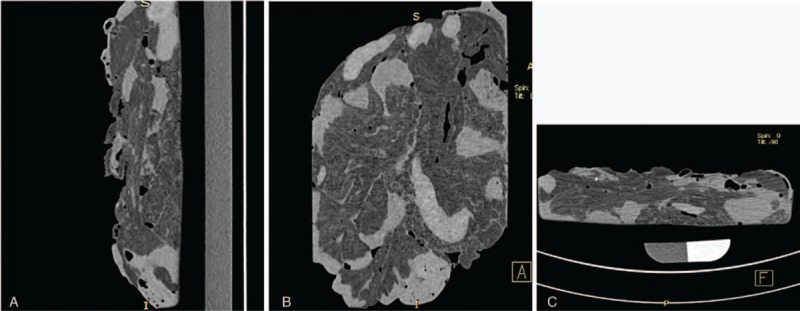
Coronal, sagittal, and cross-sectional screenshots of computed tomography images.
